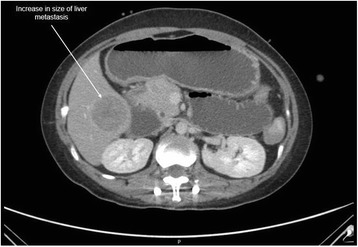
Increase in size of metastatic liver nodule on subsequent CT
